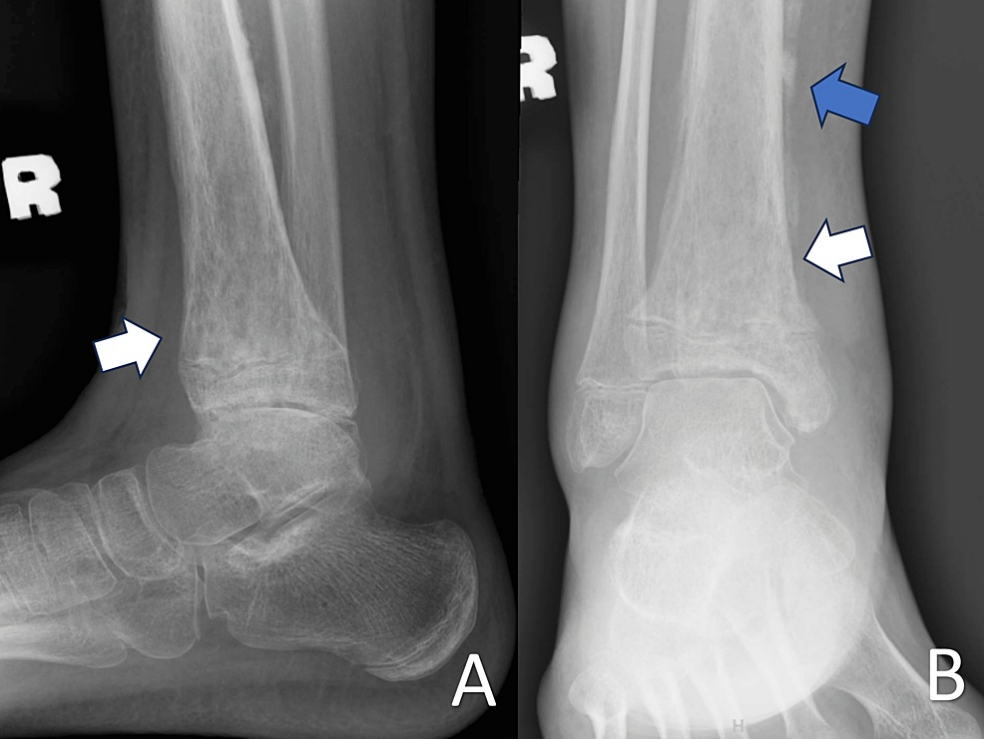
Plain radiograph of the left ankle in anteroposterior (A) and lateral (B) views The images revealed bony sclerosis (white arrows) and periosteal callus formation (blue arrow)
